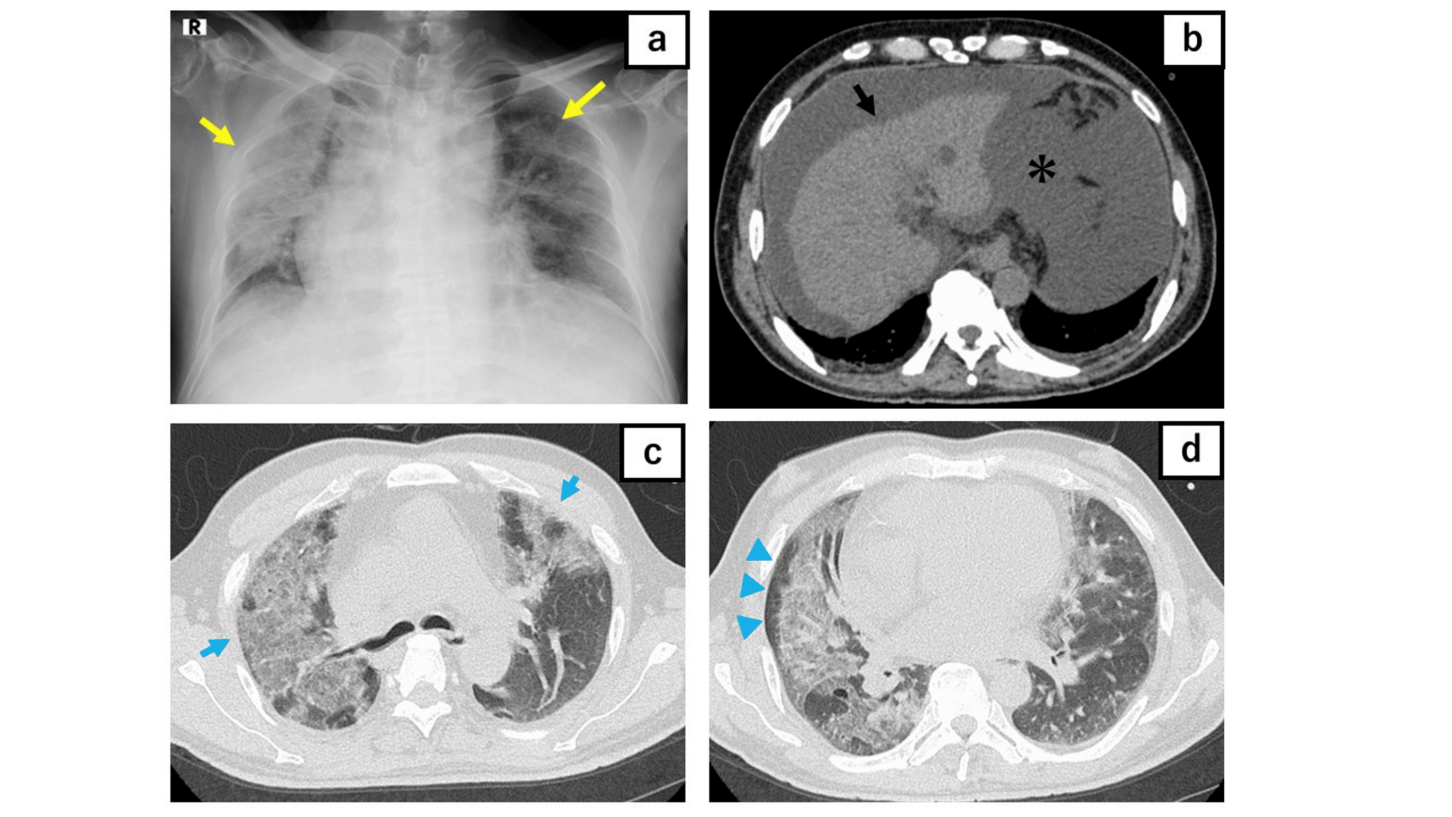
X-ray and CT images A chest X-ray showed bilateral GGOs and consolidation (yellow arrow) (a). A non-contrast abdominal CT scan showed ascites (asterisk) and liver atrophy (black arrow) (b). A chest CT scan demonstrated diffuse GGOs with a mosaic pattern (blue arrow) with subpleural sparing (blue arrowhead) (c, d).
